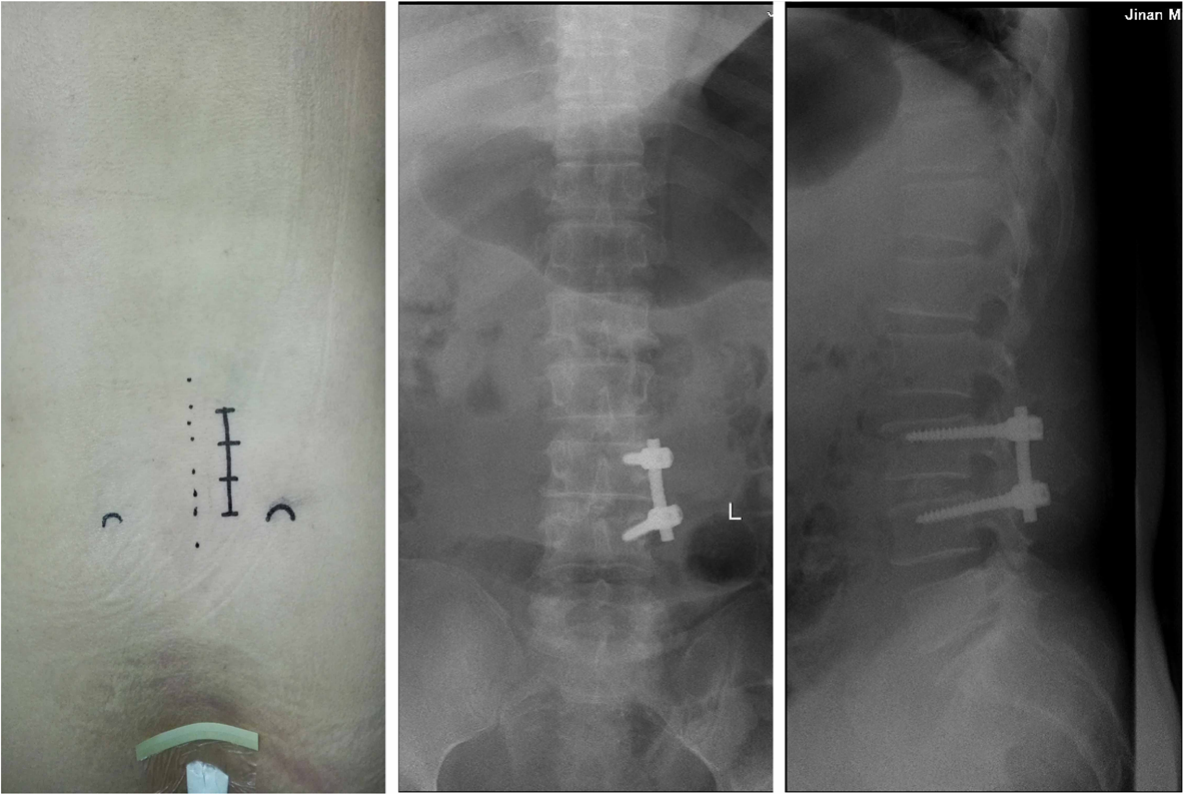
Anteroposterior and lateral CT scan showing MIS TLIF with UPS fixation in lumbar spinal fusion
